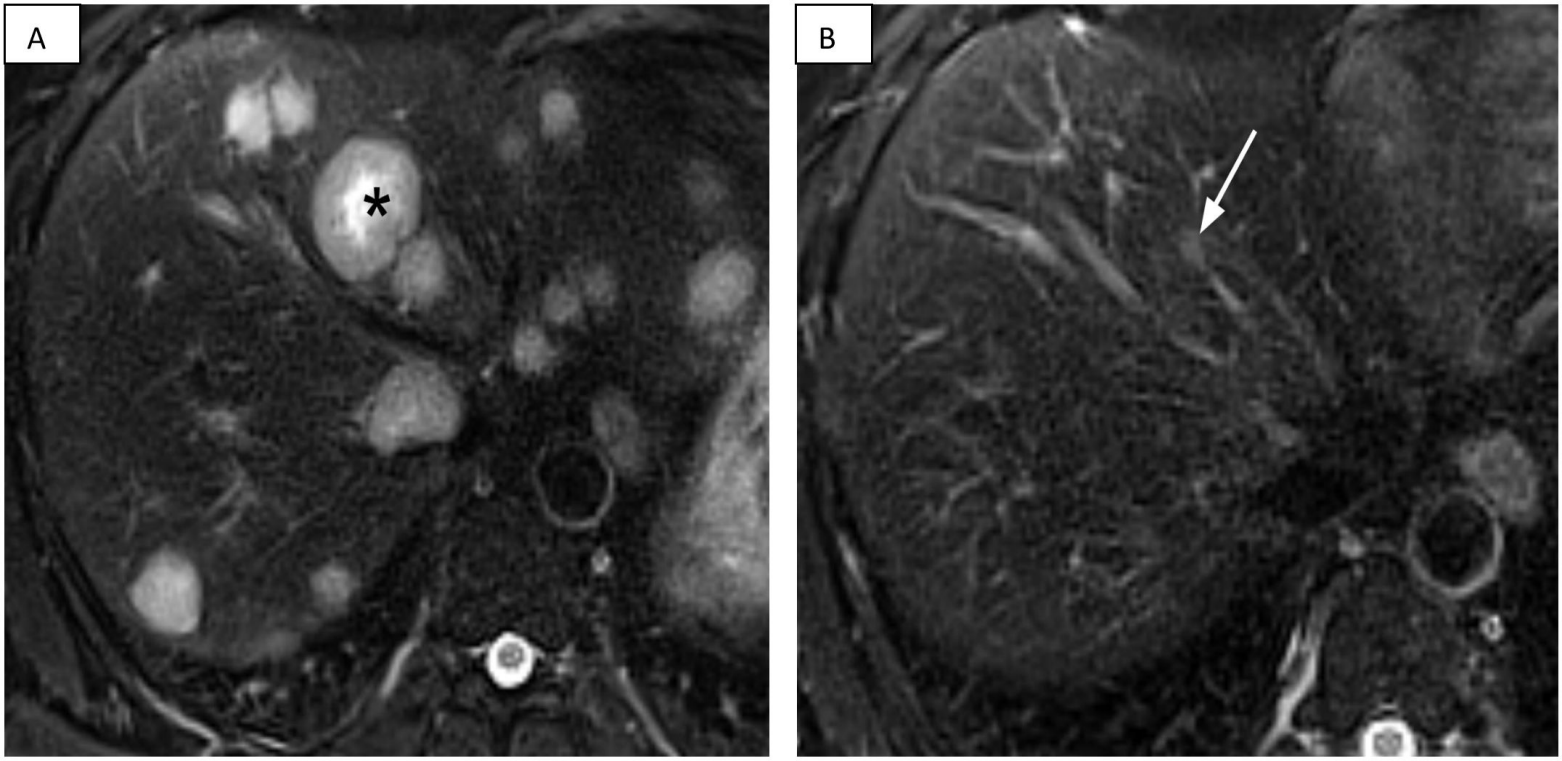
MRI of the abdomen. (A) Baseline MRI of the abdomen. Axial T2-weighted fat suppressed MRI of the abdomen at baseline (August 2024) reveals innumerable, widespread T2-hyperintense hepatic metastases involving all segments of the liver (black asterisk denotes largest lesion). (B) Follow-up MRI of the abdomen. A follow-up axial T2-weighted fat suppressed MRI of the abdomen (August 2025), after approximately 7.5 months of talazoparib, demonstrates near resolution of the hepatic metastases, with only minimal residual signal abnormality within some of the initially largest lesions (white arrow).
